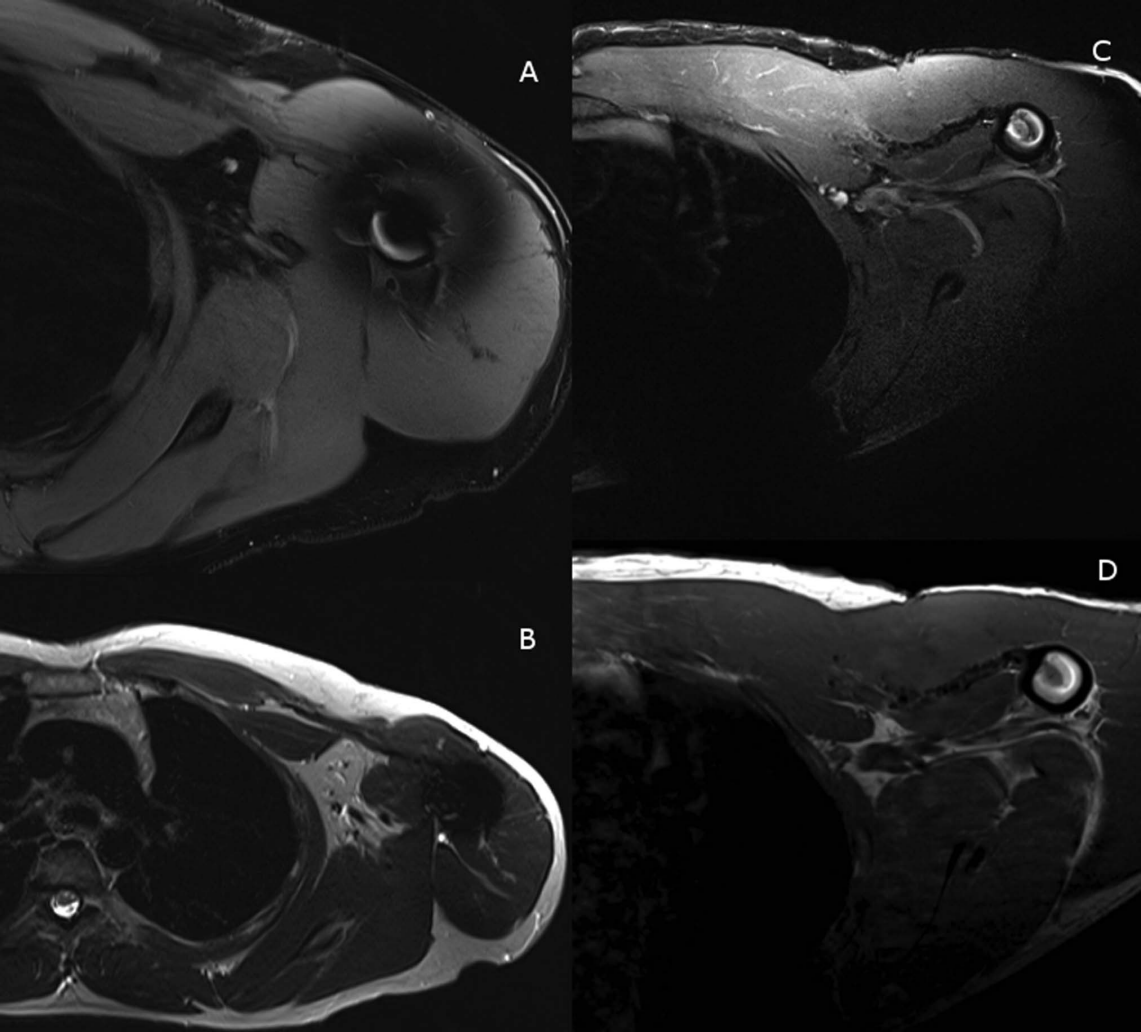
Postoperative magnetic resonance images. (A and B) Axial images for patient 1 demonstrate dermal allograft interpositional repair intact and in continuity. (C and D) Axial images for patient 2 demonstrate dermal allograft interpositional repair intact and in continuity.
